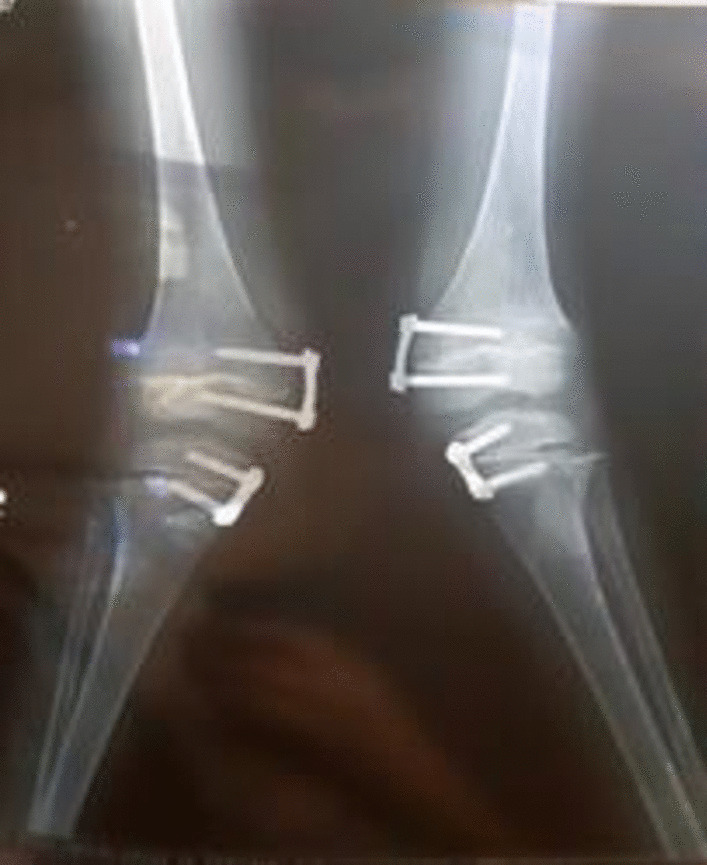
Postoperative radiograph following guided growth procedure showing improvement in the bilateral genu valgum deformity
